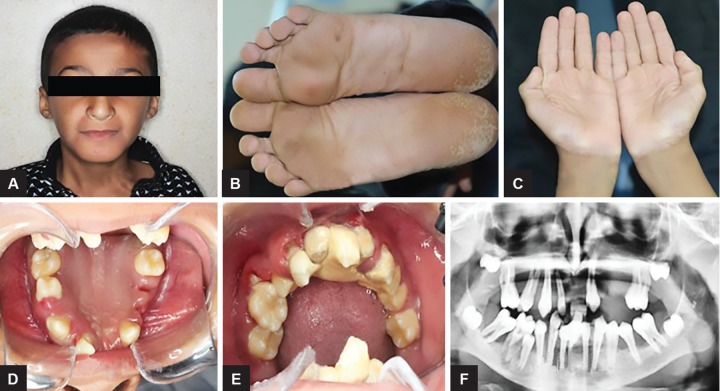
(A) An 11-year-old male patient; (B) plantar hyperkeratosis; (C) Palmar hyperkeratosis; (D) intraoral clinical picture of the maxillary arch teeth; (E) intraoral clinical picture of the mandibular arch teeth; (F) orthopantomogram showing multiple loss of teeth and the alveolar bone loss around many present teeth
